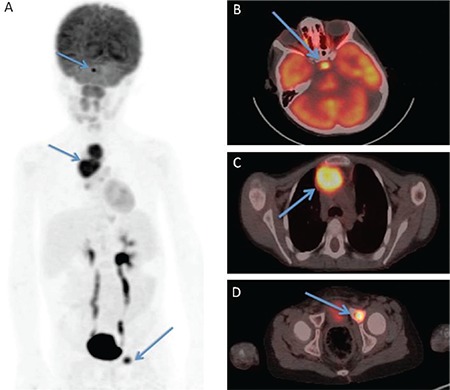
Maximum intensity projection 18F-fluorodeoxyglucose positron emission tomography images of the patient revealed pathological uptake in the cranium, thorax, and left pelvic region (a). Fused trans-axial images demonstrated pathological uptakes, located in the pituitary gland (SUV_max_: 9.8) (b), soft tissues in the anterior mediastinum (SUV_max_: 10.7) (c), and the left acetabular region (SUV_max_: 10.6) (d).
